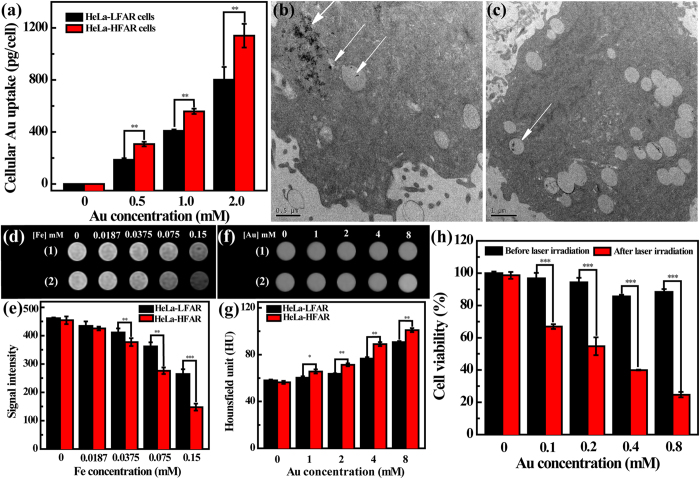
In vitro* cellular uptake assay, targeted MR and CT imaging and photothermal ablation of HeLa cells. (a) The Au uptake by HeLa-HFAR and HeLa-LFAR cells after treated with the Fe_3_O_4_ @ Au-PEI.Ac-PEG-FA NSs at various Au concentrations for 4 h. TEM images of (b) HeLa-HFAR and (c) HeLa-LFAR cells after treated with the Fe_3_O_4_ @ Au-PEI.Ac-PEG-FA NSs for 4 h, respectively. (d) T_2_-weighted MR images, (e) MR signal intensity, (f) CT images, and (g) CT value of HeLa-HFAR and HeLa-LFAR cells treated with the Fe_3_O_4_ @ Au-PEI.Ac-PEG-FA NSs at varying Fe or Au concentrations for 6 h. (h) MTT assay of HeLa cell viability after treatment with the Fe_3_O_4_ @ Au-PEI.Ac-PEG-FA NSs in a given Au concentration range under an 808 nm laser irradiation for 5 min. 1 and 2 represent the HeLa-LFAR and HeLa-HFAR cells, respectively.
